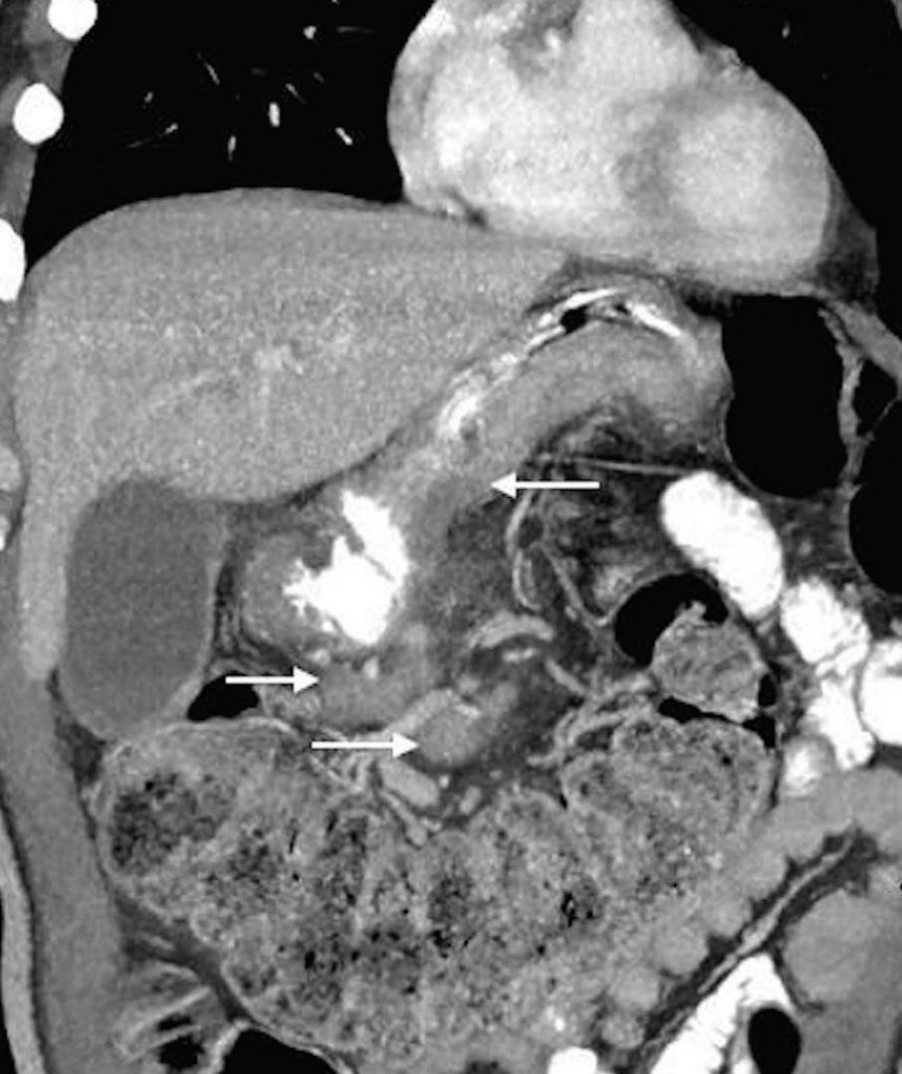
Coronal oblique 5 mm MIP reconstruction CT image from Patient 1 before treatment. This image shows asymmetric, diffuse thickening of the inferior wall of gastric body and antrum with infiltration of adjacent fat as well as extensive mesenteric lymphadenopathy.
